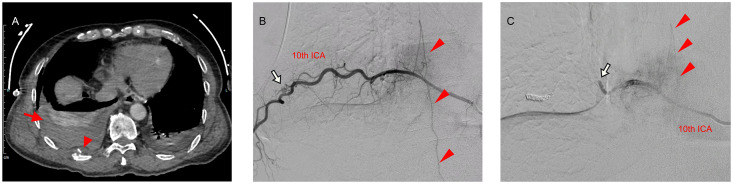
(A) Contrast-enhanced computed tomography (CT) obtained 3 days post-injury demonstrated a hemothorax (arrow) and a rib fracture (arrowhead) with no contrast medium extravasation. (B) Selective angiography of the right 10th intercostal artery revealed a small pseudoaneurysm (arrow) in the distal segment at the rib fracture level. The Adamkiewicz artery (arrowheads) originates from the proximal portion of the artery. (C) Embolization using metallic coils was successfully performed. Angiography after embolization demonstrated complete occlusion of the artery (arrow). The Adamkiewicz artery (arrowheads) is patent.
